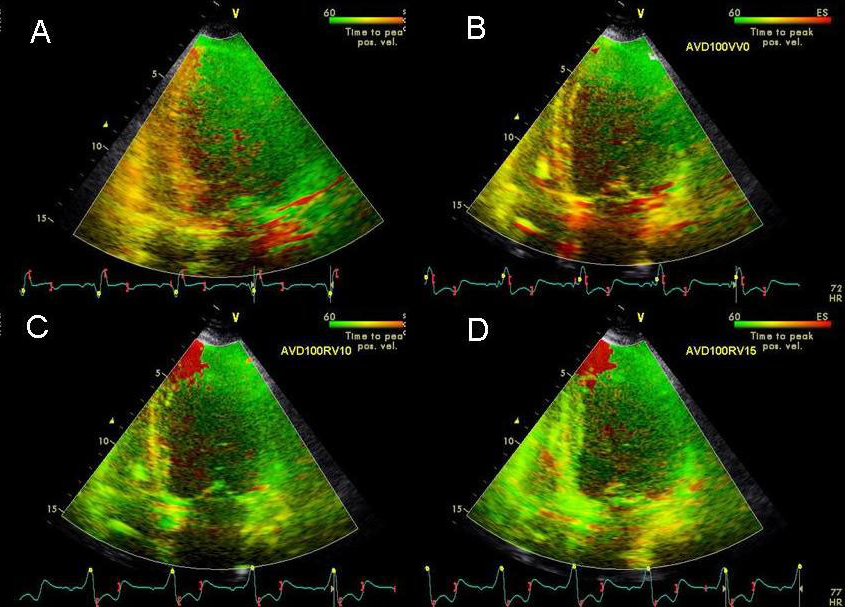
TSI in the apical 4 chamber views showing the effect of right ventricular pre-excitation on interventricular septal and right ventricular free wall delay. At baseline with LV pre-excitation of 20 ms there is marked delay in interventricular septum and RV free wall shown in orange color in A. VV of 0 ms reduces this interventricular septal and RV free wall delay (B). RV pre-excitation of 10 ms improves this further (C) and RV pre-excitation of 15 ms abolishes remaining mild delay in the interventricular septum (D). There is appearance of a small area of delay in the left ventricular apex (shown in red in C and D) with RV pre-excitation. There was progressive improvement in LV strain with RV pre-excitation as shown in Figure 11.
